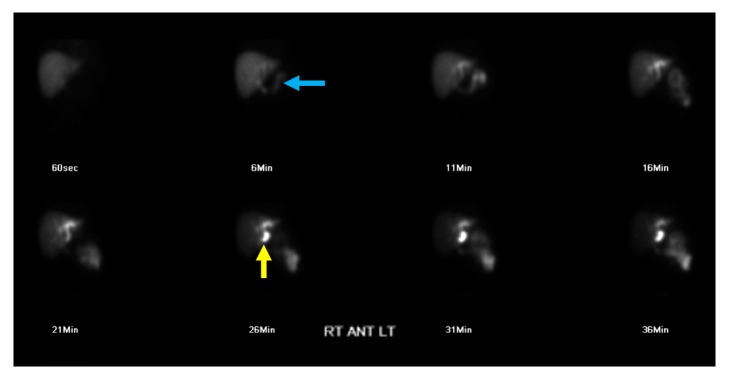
22-year-old male with history of CF presents with recurrent bouts of sharp RUQ abdominal pain. Findings: hepatobiliary scintigraphy HIDA scan demonstrating bowel activity (blue arrow) at 6 minutes and gallbladder activity (yellow arrow) at 26 minutes from the time of tracer intravenous injection of 3.3 mCi of Technetium 99m Choletec.
